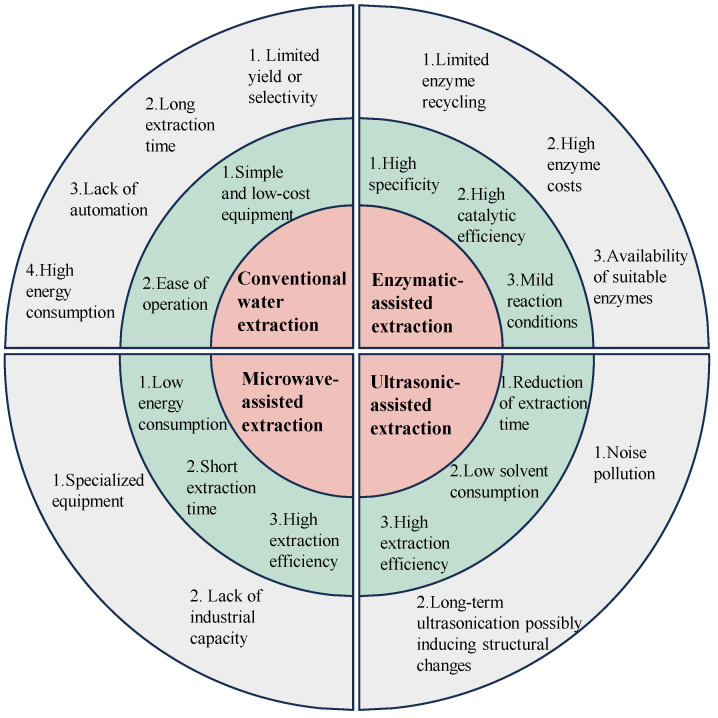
The pros and cons of the conventional extraction method and advanced extraction techniques, including microwave-assisted, ultrasonic-assisted, and enzymatic-assisted extraction. Red indicates the extraction methods, green highlights the advantages of each extraction method, and grey points out the disadvantages of each extraction method.
